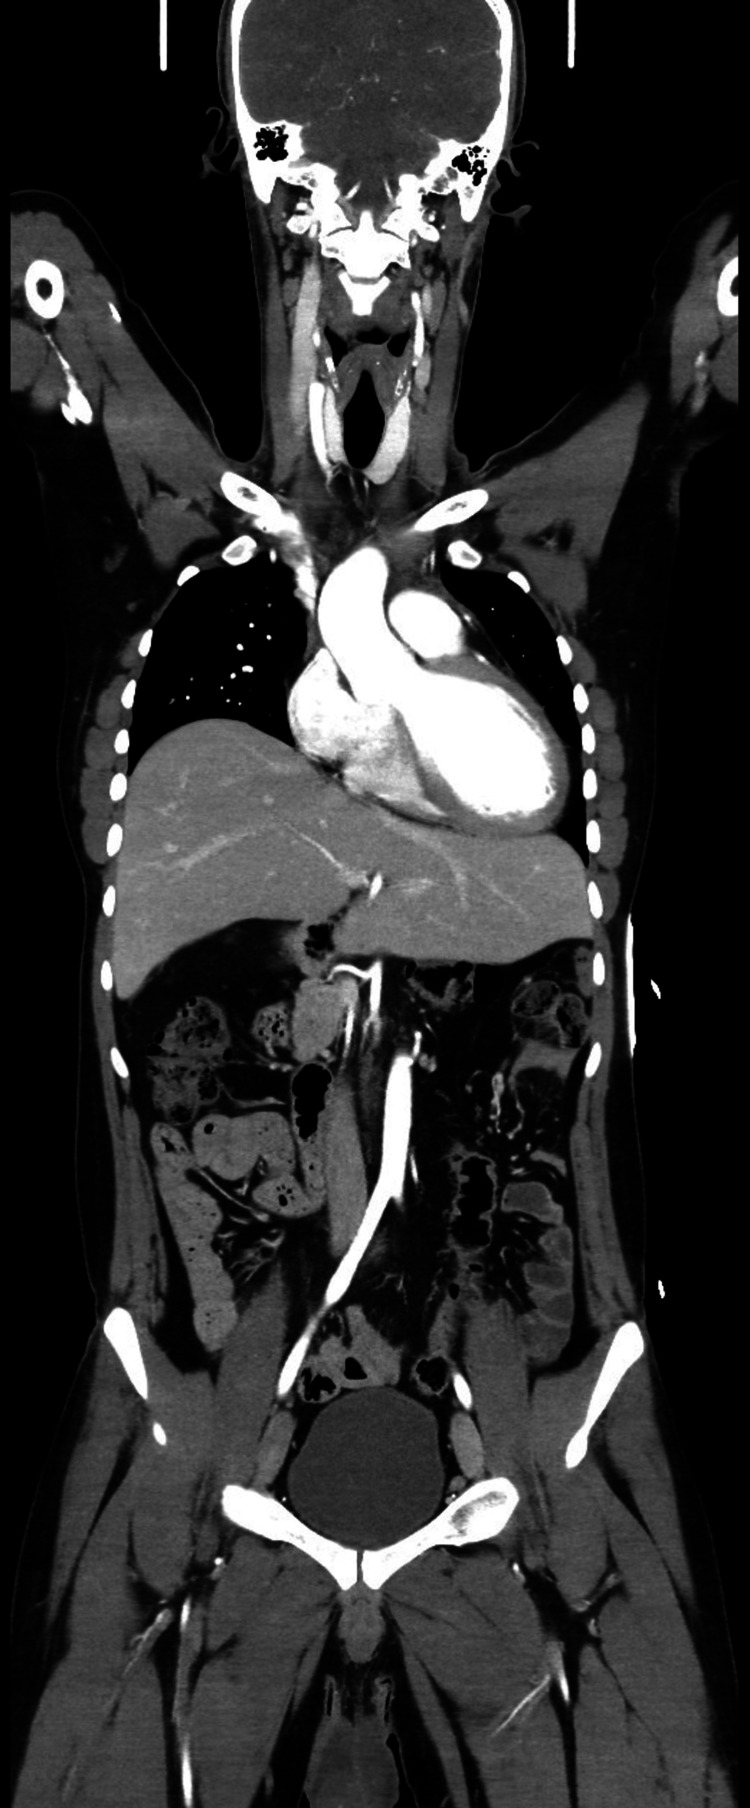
Contrast-enhanced CT demonstrating no evidence of acute traumatic injury to the brain, skull, spine, thorax, pelvis, or solid organs in the abdomen. No free fluid, hemoperitoneum, pneumothorax, or visceral laceration is identified.
